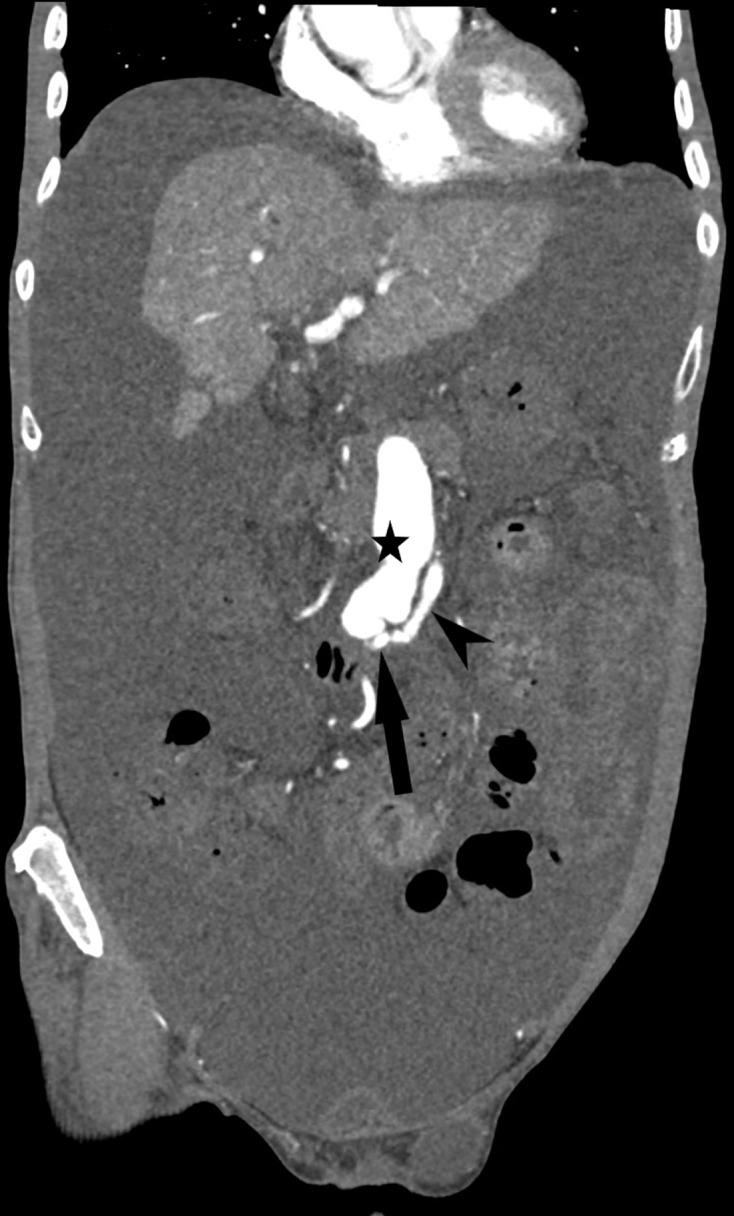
Contrast-enhanced CT with coronal reconstruction (arterial phase) shows a fistula (arrow) between superior mesenteric artery (arrowhead) and grossly distended superior mesenteric vein (star).
